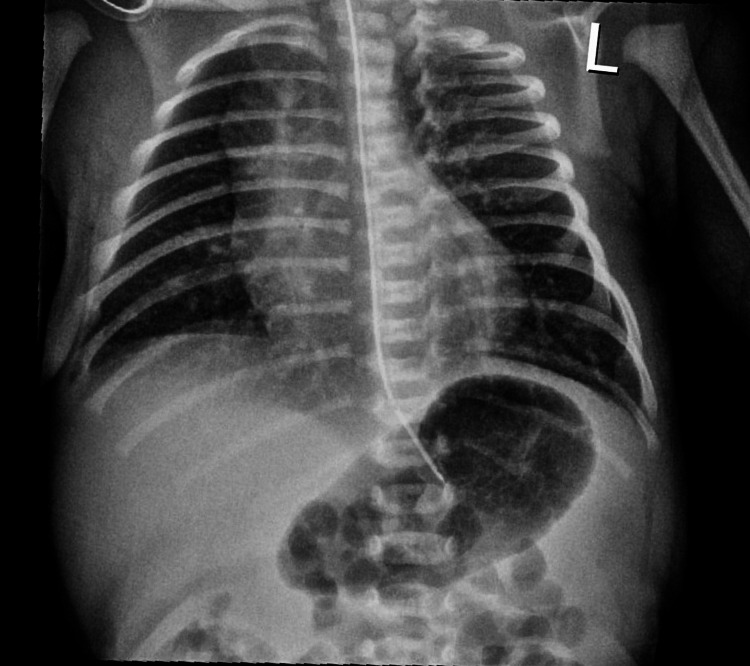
Portable frontal chest radiograph showing normal mediastinum, cardiothymic silhouette, and symmetric lung volumes. No diaphragmatic hernia seen.
